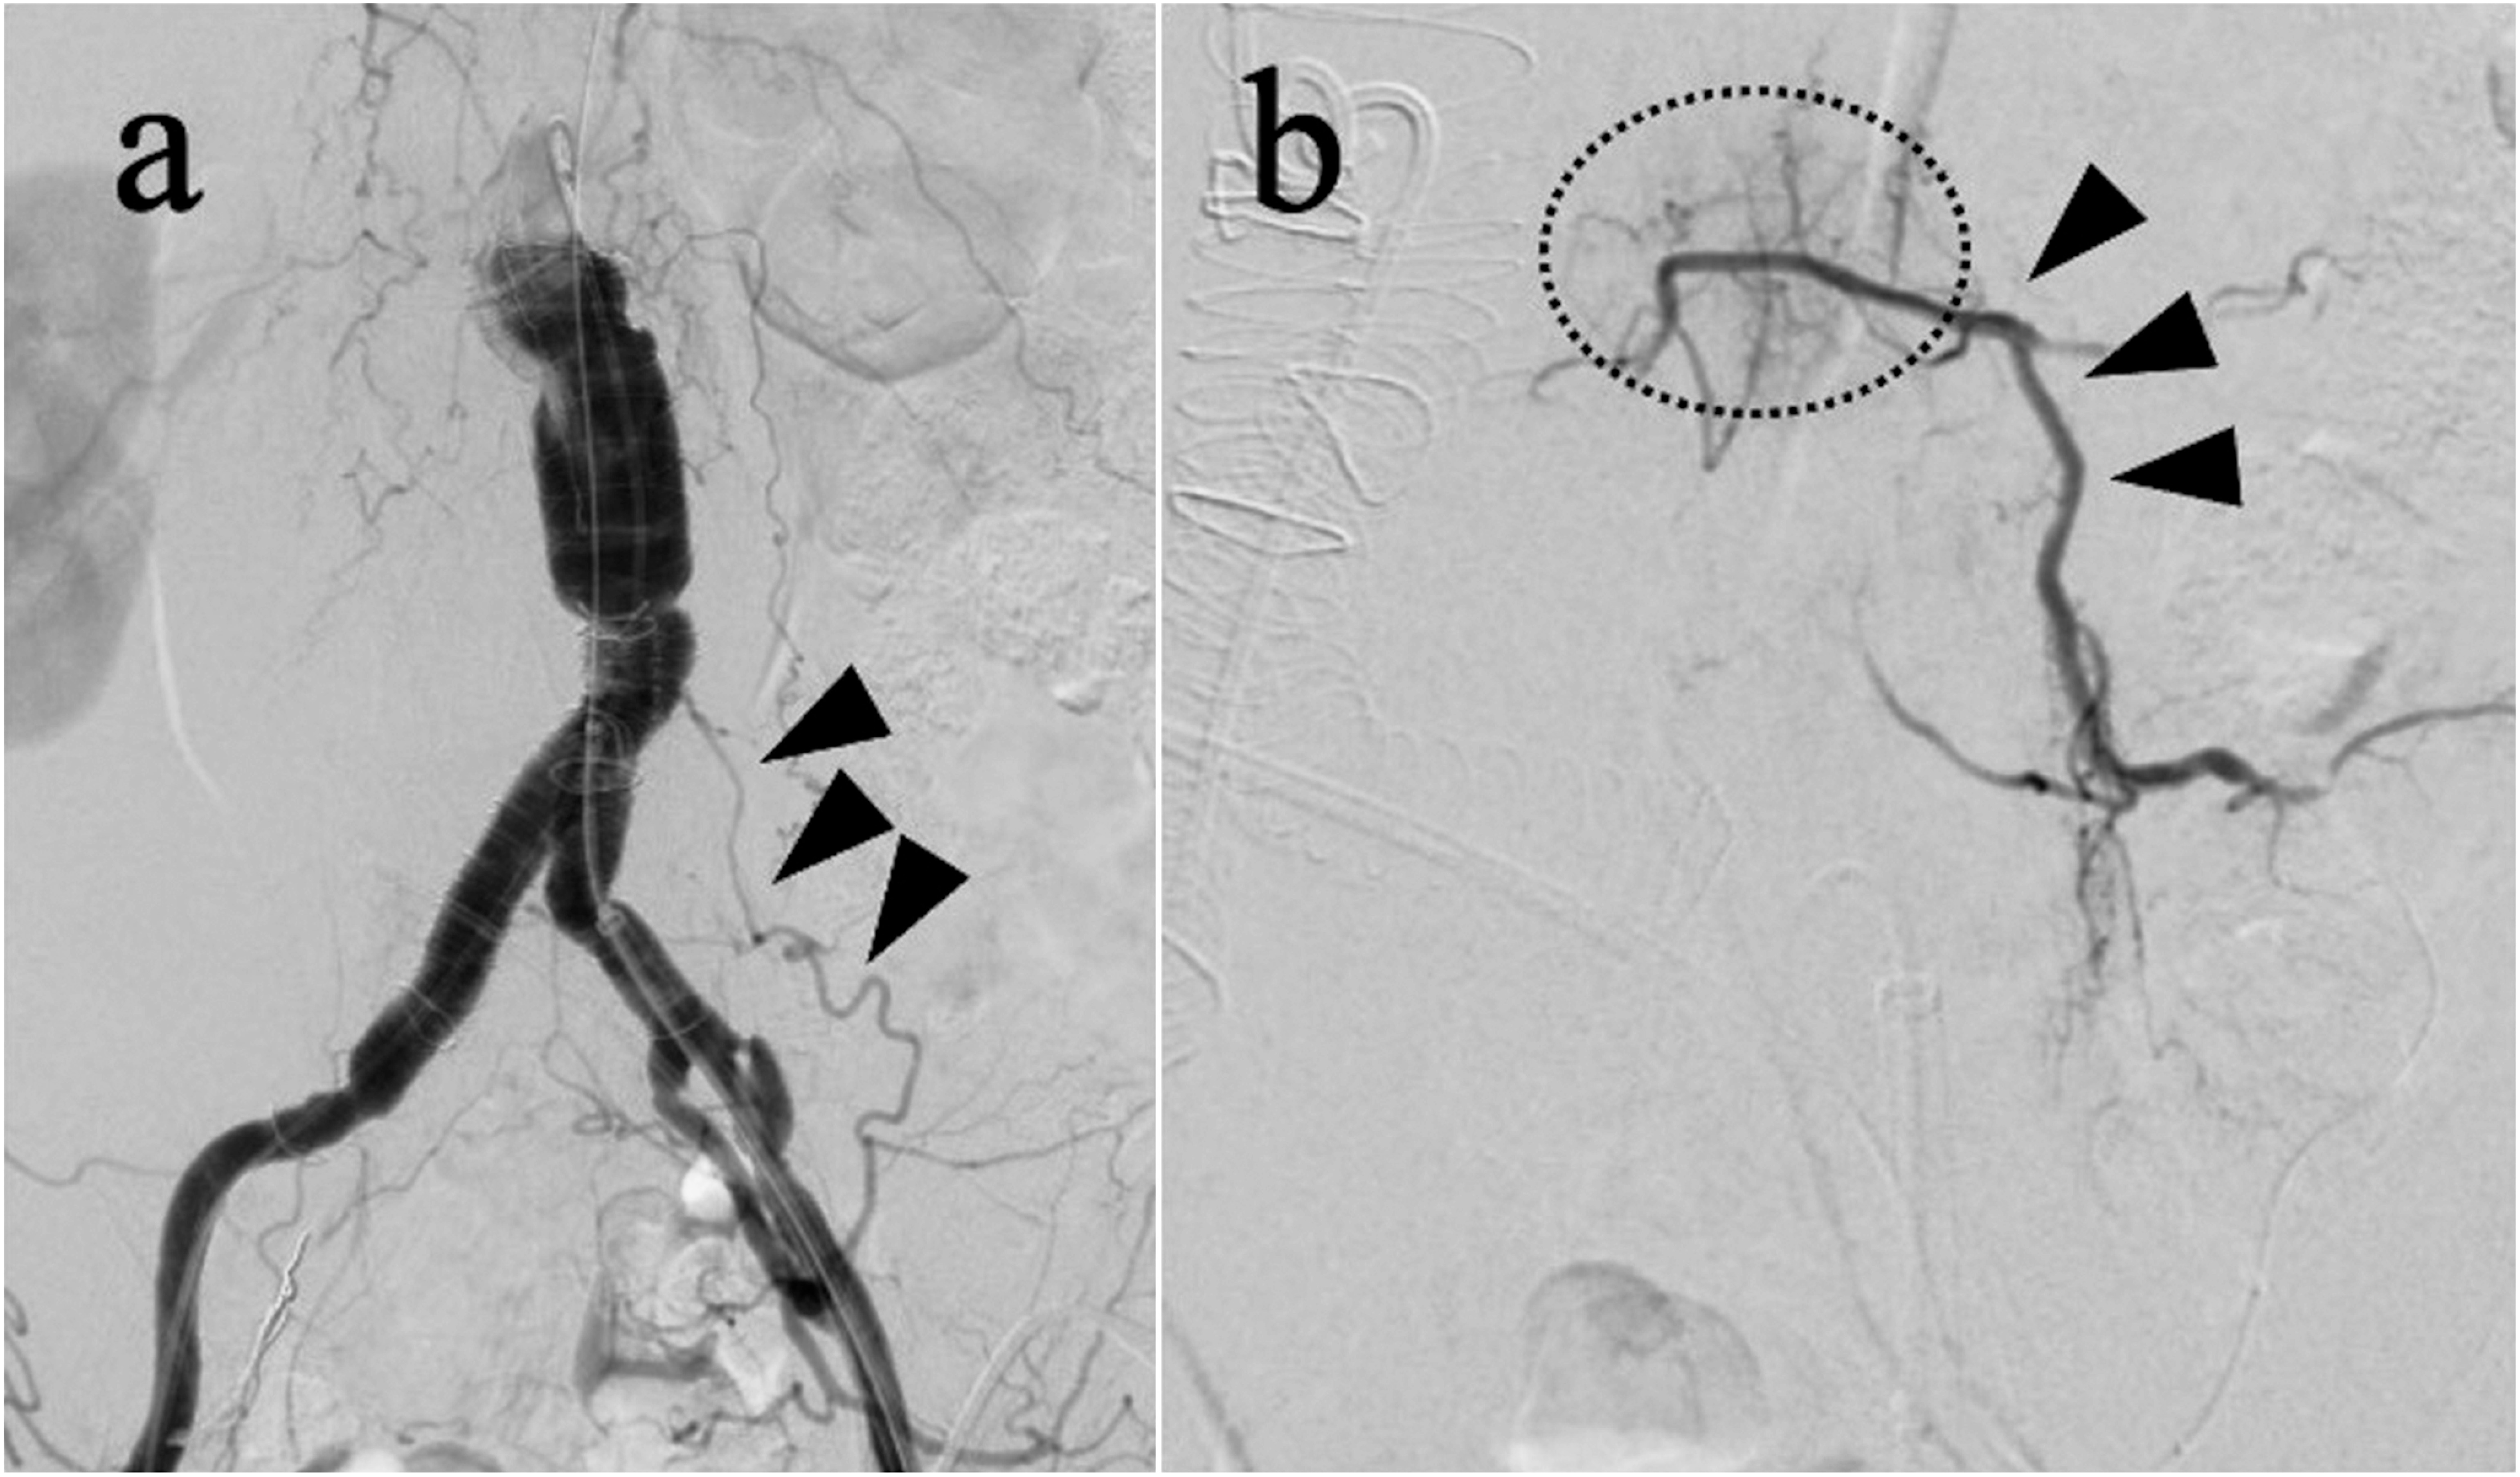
Fig. 2 (a) Intraoperative angiography reveals no endoleaks of any type that can cause aneurysm rupture (arrowheads). (b) The lumbar artery causing the bleeding in the retroperitoneal space is embolized using lipiodol (arrowheads). As in the CT, extravasation is detected in the lumbar artery at the level of the fourth lumbar vertebra (black circle).
